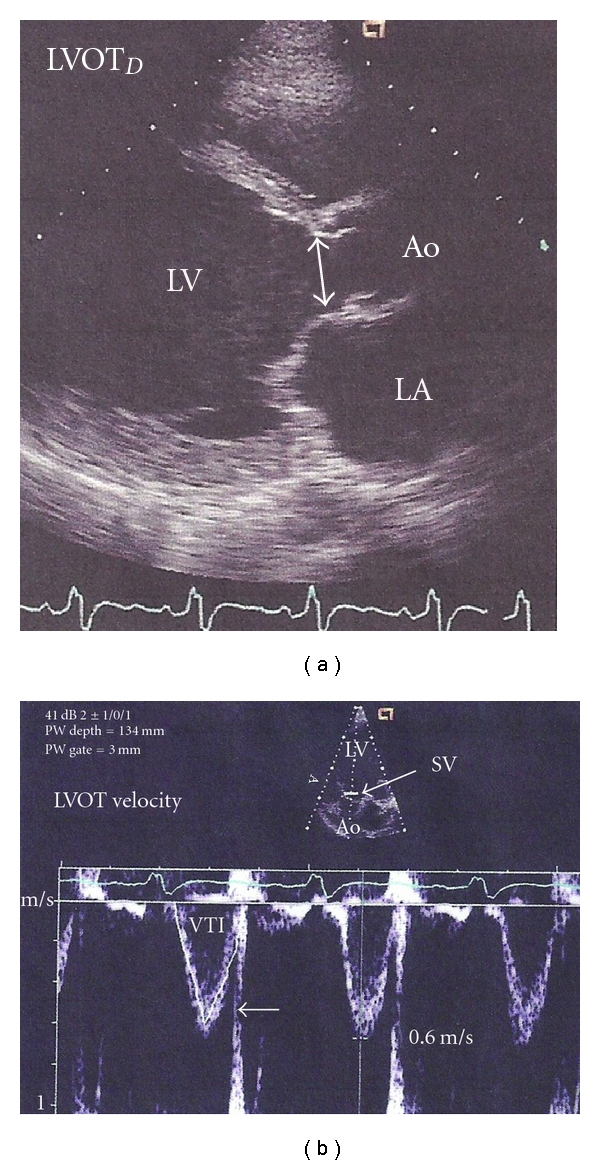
Transducer placement and characteristic waveform using transcutaneous doppler ultrasonography. This demonstrates the characteristic transducer direction and image capture using the direct measurement through the left ventricular outflow tract. One notes a characterstic waveform and manipulates the transducer to obtain maximum outflow. This figure was published in the Textbook of Clinical Echocardiography, 3rd edition, Elsevier, Ltd, 2004. Page 148, Used with permission [25].
